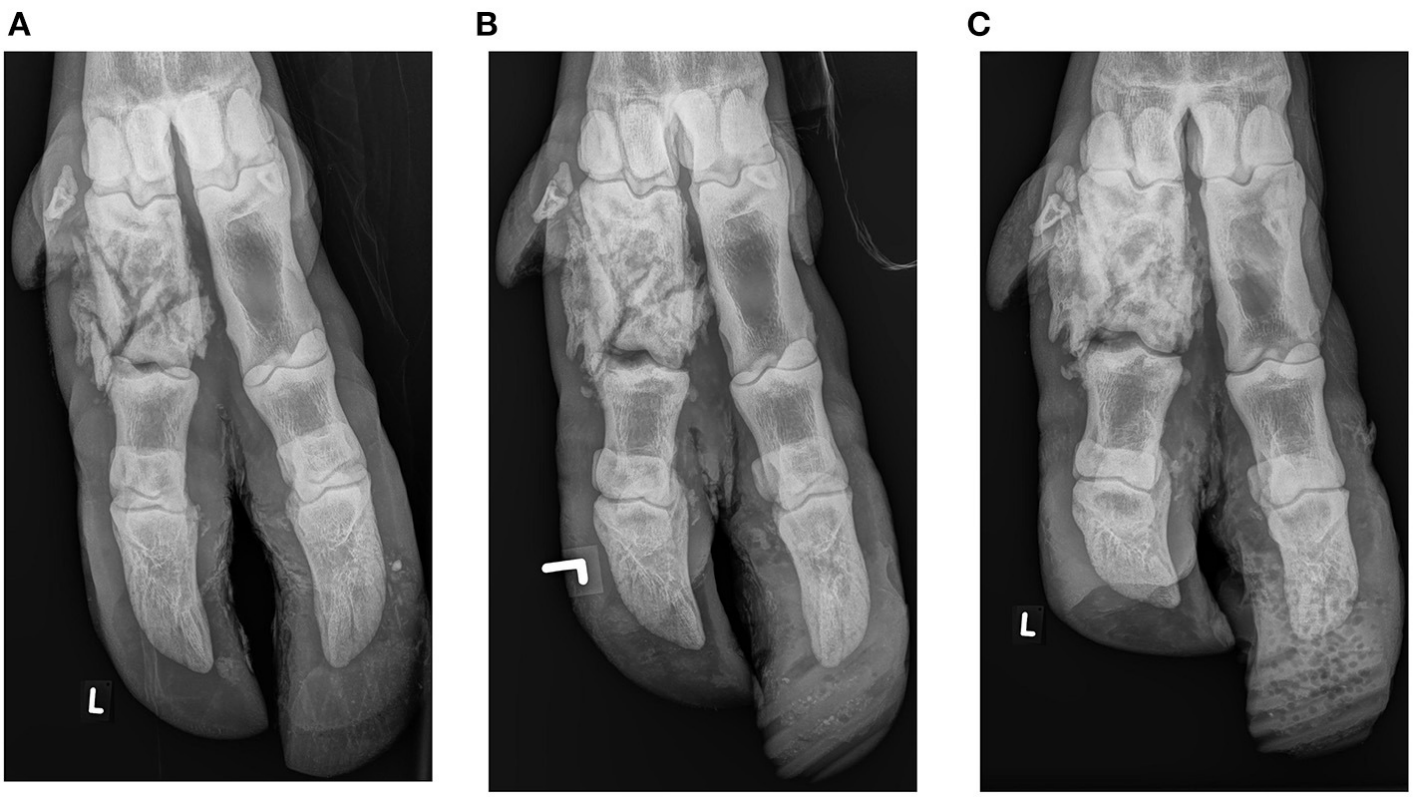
Plantarodorsal radiographs of a multifragmentary fracture of the lateral proximal phalanx in an adult female European bison (*Bison bonasus*): Six (A), 9 (B), and 11 weeks (C) after the injury. At the initital treatment, a wooden claw block was used and polyurethane blocks thereafter.
